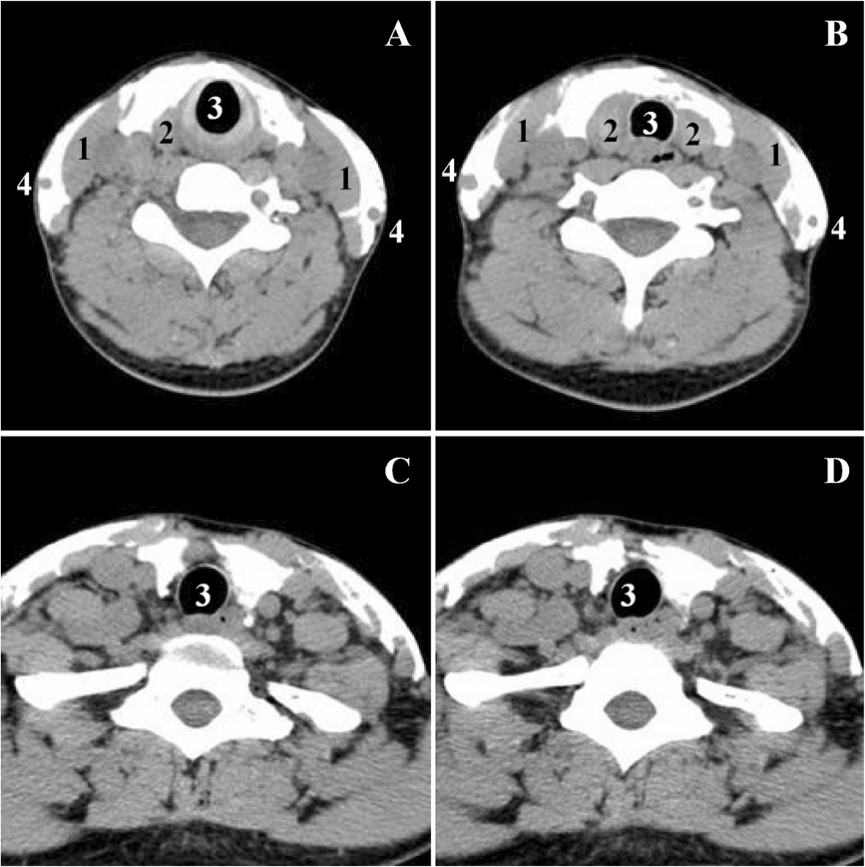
Computed tomography (CT) images of spreading patterns of the contrast medium in ultrasound-guided capsule-sheath space block (CSSB) combined with anterior cervical cutaneous nerves block (CCNB). CT scan was respectively obtained at the level of upper pole (A), middle of the lobes (B), lower pole (C), and cervical region VI (D) in a 35-yr-old female volunteer. It demonstrated that the injectate spread along bilateral SCM muscles in superficial compartment. Likewise, the injectate in capsule-sheath space could spread up to the upper pole and down to the lower pole and even lower region VI. Furthermore, contrast medium reached the posterior aspect of the two poles. 1, sternocleidomastoid muscle; 2, thyroid gland; 3, trachea; 4, external jugular vein.
